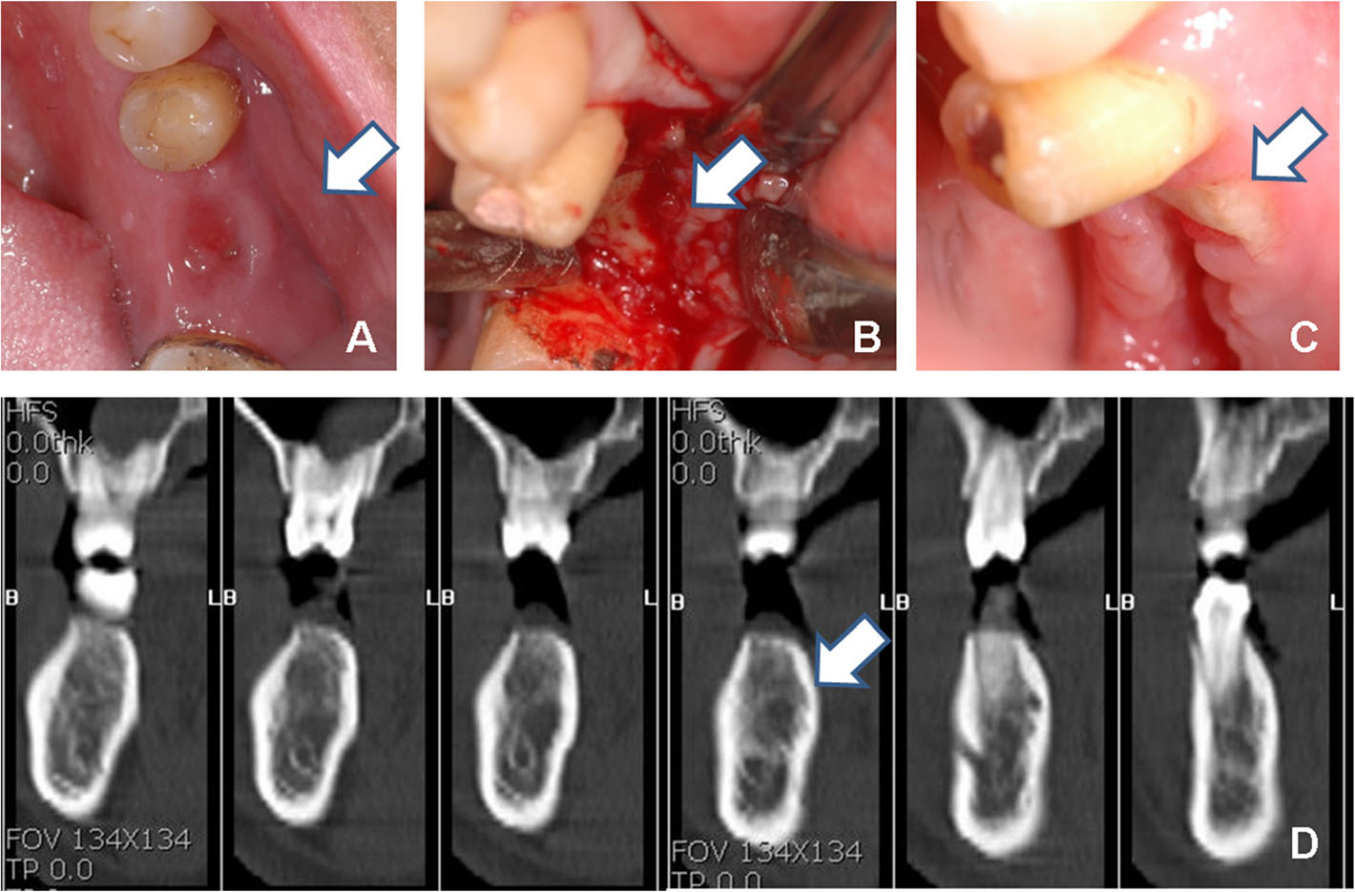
Clinical features and CT of MRONJ patient. a Mucosal fistula on the pontic area. b Intraoperative findings during bone biopsy. c Exposed alveolar bone. d CT findings
